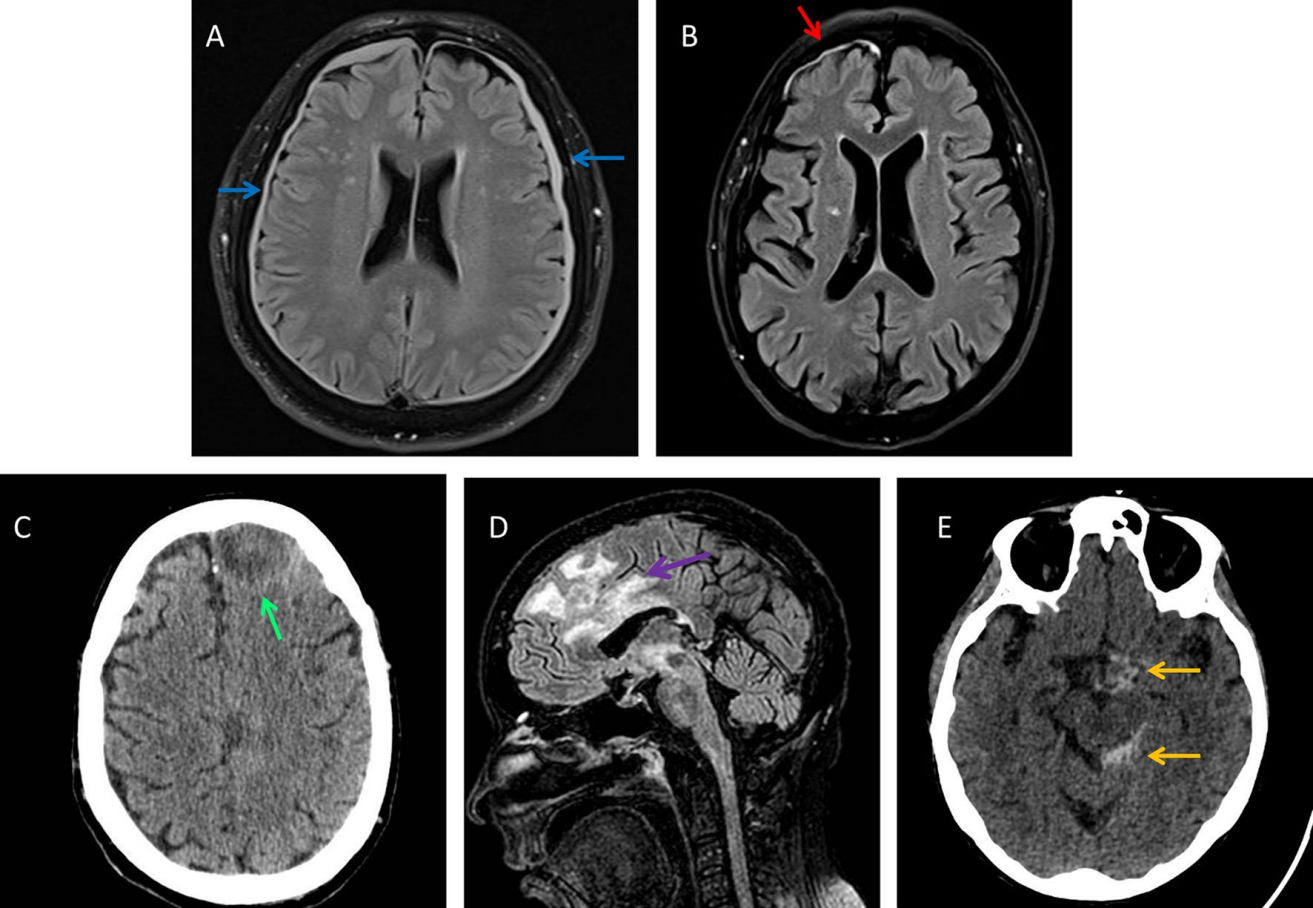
A Brain MRI documents bilateral subdural hematoma (blue arrows) in the frontal and parietal lobe (4–5 mm maximum thickness) with a thin and diffuse dural hyperintense FLAIR signal. B Frontal right subdural effusion (9 mm thick, red arrow) shows hyperintense FLAIR signal and heterogeneous but largely hyperintense T1 signal (not shown); meninges in that site show intense contrast enhancement (not shown). These findings could be referred to a chronic subdural hematoma with signs of more recent bleeding. C CT scan shows a heterogeneous and blurred hypodense lesion with a rim-like hyperdense tissue (green arrow) in the left paramedian frontal lobe (about 3.8 × 2.8 cm). D MRI of the sagittal plane documenting an expansive lesion (35 × 20 mm maximum) mainly in thalamic region with irregular margins (purple arrow), hyperintense FLAIR signal; the lesion extends caudally up to the homolateral midbrain and determines edematous-malacic effects up to the pons (more to the right), significant compressive phenomena on the lateral ventricle and initial contralateral midline shift (not shown). The picture is to be related to likely hemorrhagic-expansive lesion. E CT scan shows two hyperdense zones of subdural hemorrhage contouring the left perimesencephalic cisterns and the suprasellar cistern (orange arrows)
